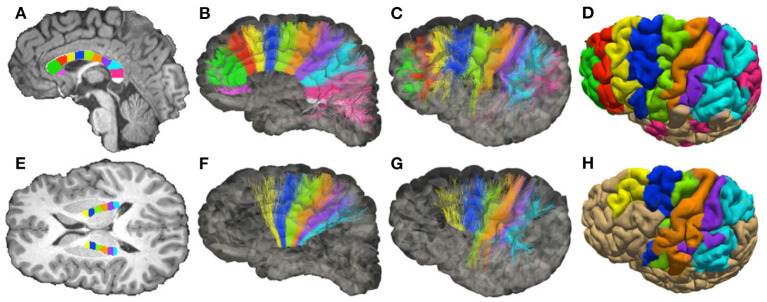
Topology of fibers in the Corpus Callosum (CC) and posterior limb of the internal capsule (PIC), reconstructed with DW-MRI tractography of a single healthy volunteer. (A) Subdivision of the mid-sagittal section of the CC in 11 sectors (corresponding to ROIs), see Supplementary Figure 9. (B,C) Streamlines colored according to the corresponding ROIs (medial and lateral views of the hemisphere). (D) Projection of the streamlines onto the pial surface. (E) Subdivision of PIC in 6 sectors (ROIs). (F,G) Streamlines colored according to the corresponding ROIs (medial and lateral view of the hemisphere). (H) Projection of the streamlines onto the pial surface.
